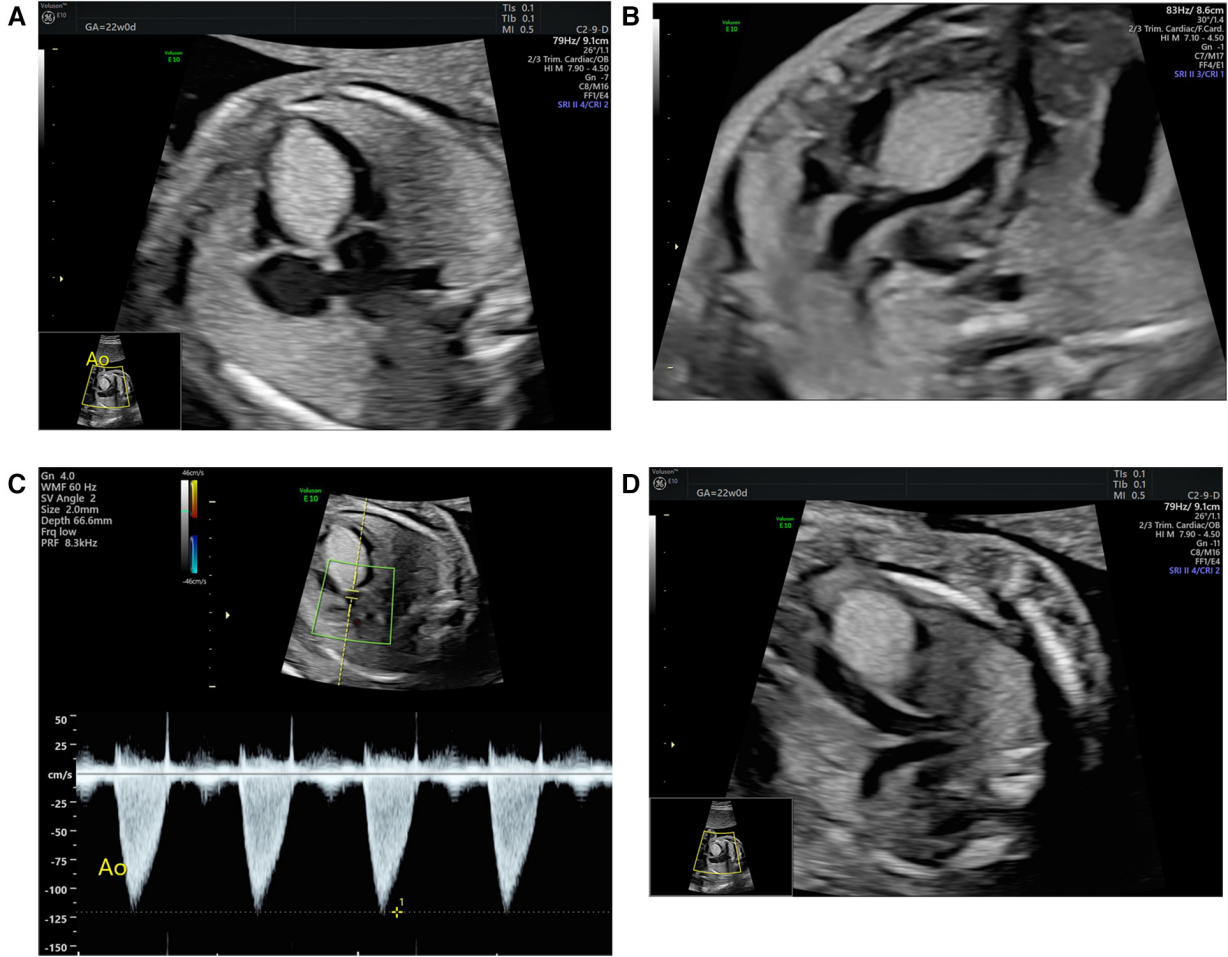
Fetal echocardiography before transplacental sirolimus therapy initiation: apical four-chamber view displaying the large hyperechogenic tumor mass (yellow markers) located in the interventricular septum, extending from the atrioventricular region to the apex and reaching into both chamber cavities (A). The modified five-chamber view (B) displays the imminent obstruction of the left ventricular outflow tract by the tumor mass, with a respectively altered Doppler flow pattern across the left ventricular outflow tract showing moderately increased flow velocity (C). The modified vessel plane (D) shows the imminent obstruction of also the right ventricular outflow tract by the tumor mass.
